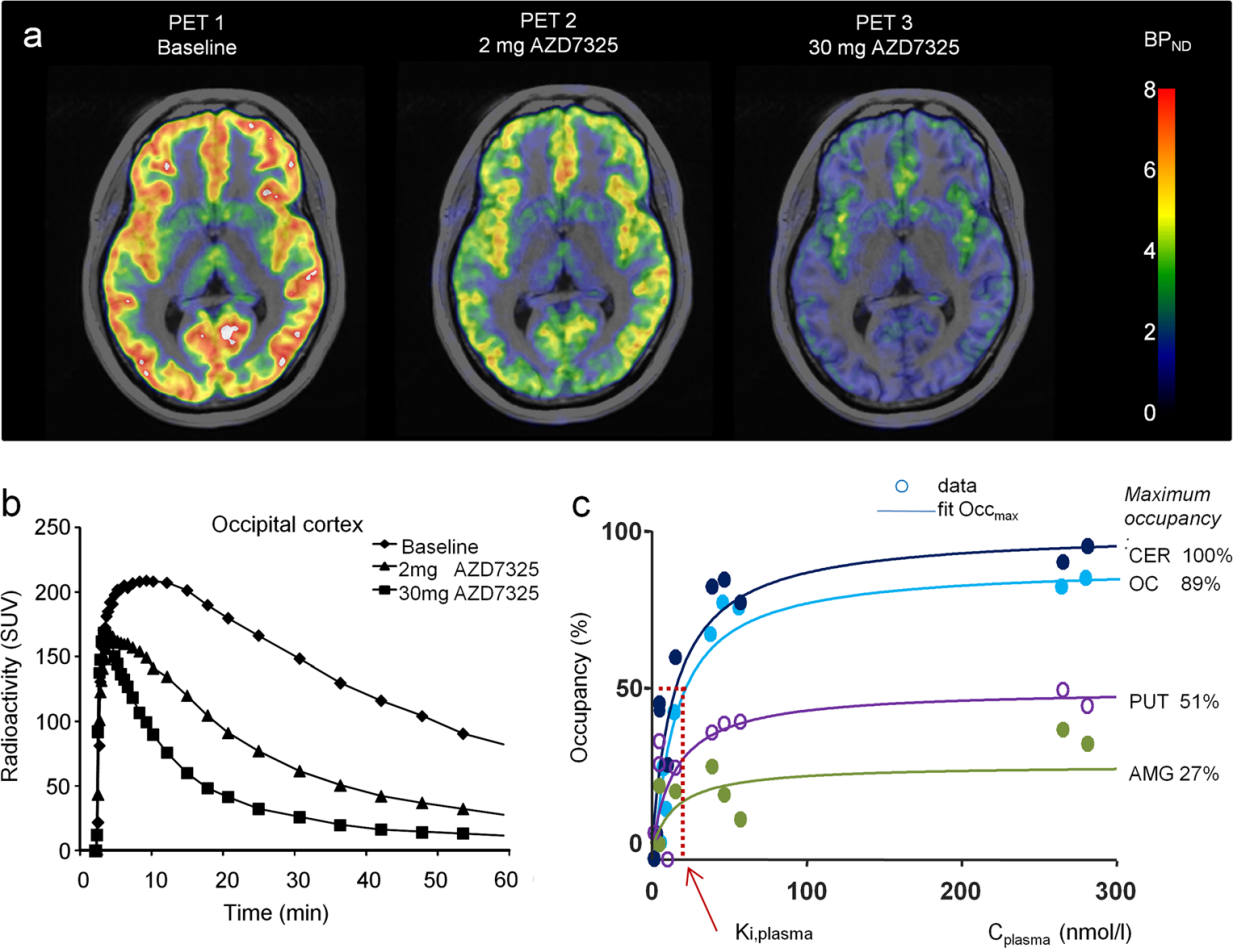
a Parametric PET images of [^11^C]flumazenil binding to brain GABA_A_ receptors at baseline and after oral administration of 2 and 30 mg of AZD7325 (PET images obtained using wavelet-aided parametric mapping (Cselényi et al. 2002) and fused with MR images; *BP*
